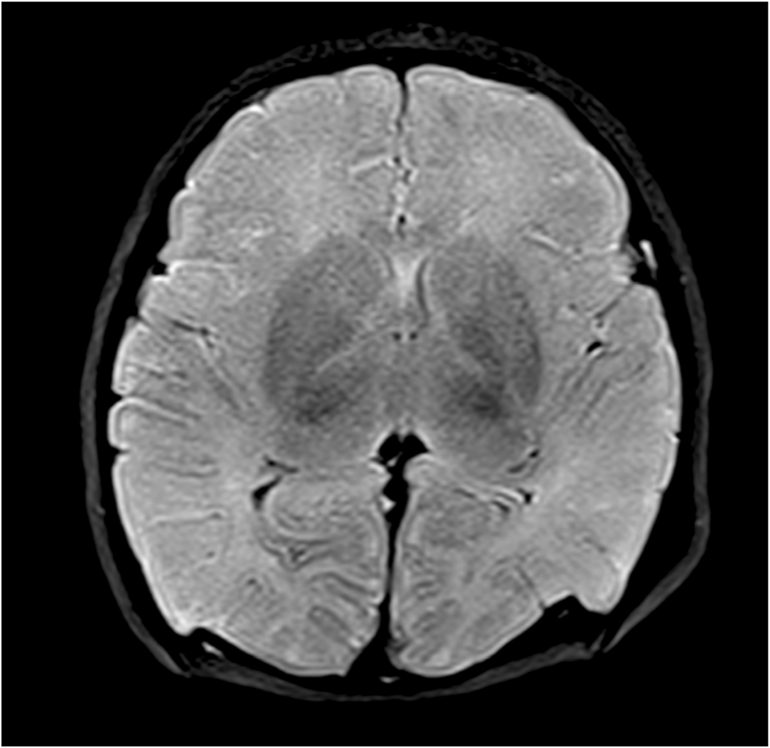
PMMR of hypoxic brain changes. Axial T2-weighted PMMR image through a fetal post mortem brain, showing an example of typical low signal change in the basal ganglia which may be associated with hypoxia. Conventional PMMR cannot currently distinguish antemortem from postmortem hypoxic change
